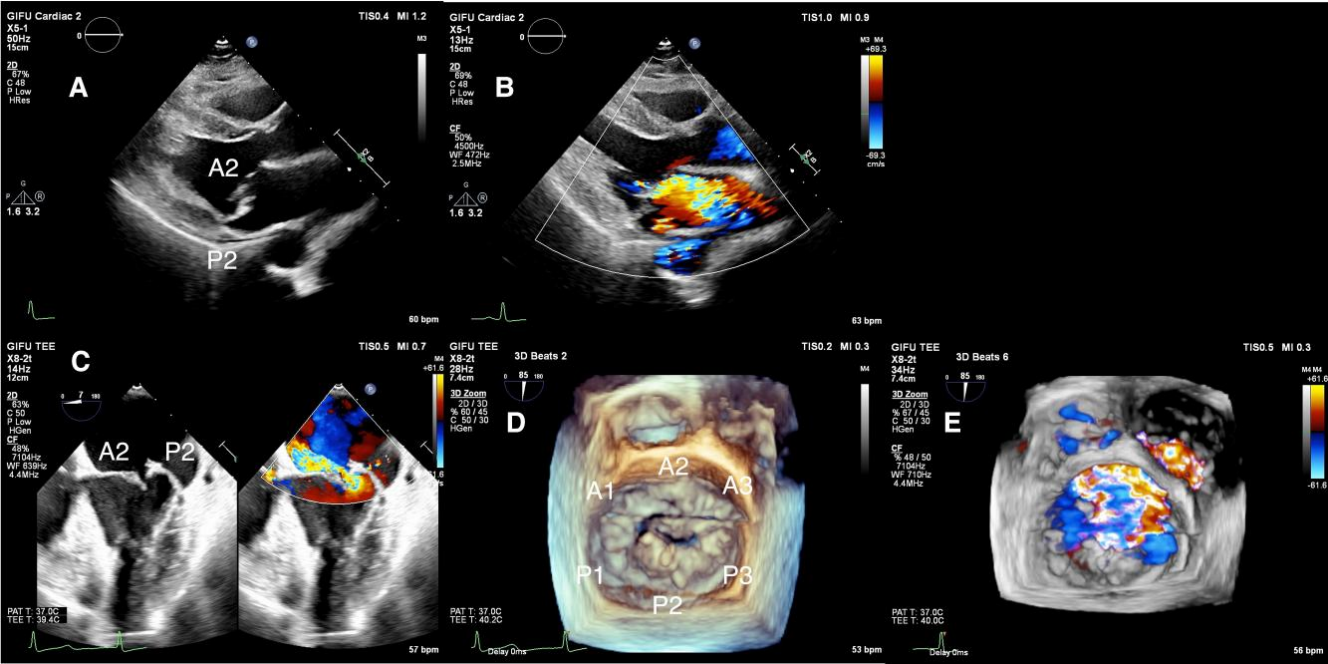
Echocardiography before mitral valve transcatheter edge-to-edge repair (M-TEER). Recurrent P2 prolapse and severe mitral regurgitation by parasternal long-axis views (*A*, *B*) and by transoesophageal echocardiography (*C–E*).
